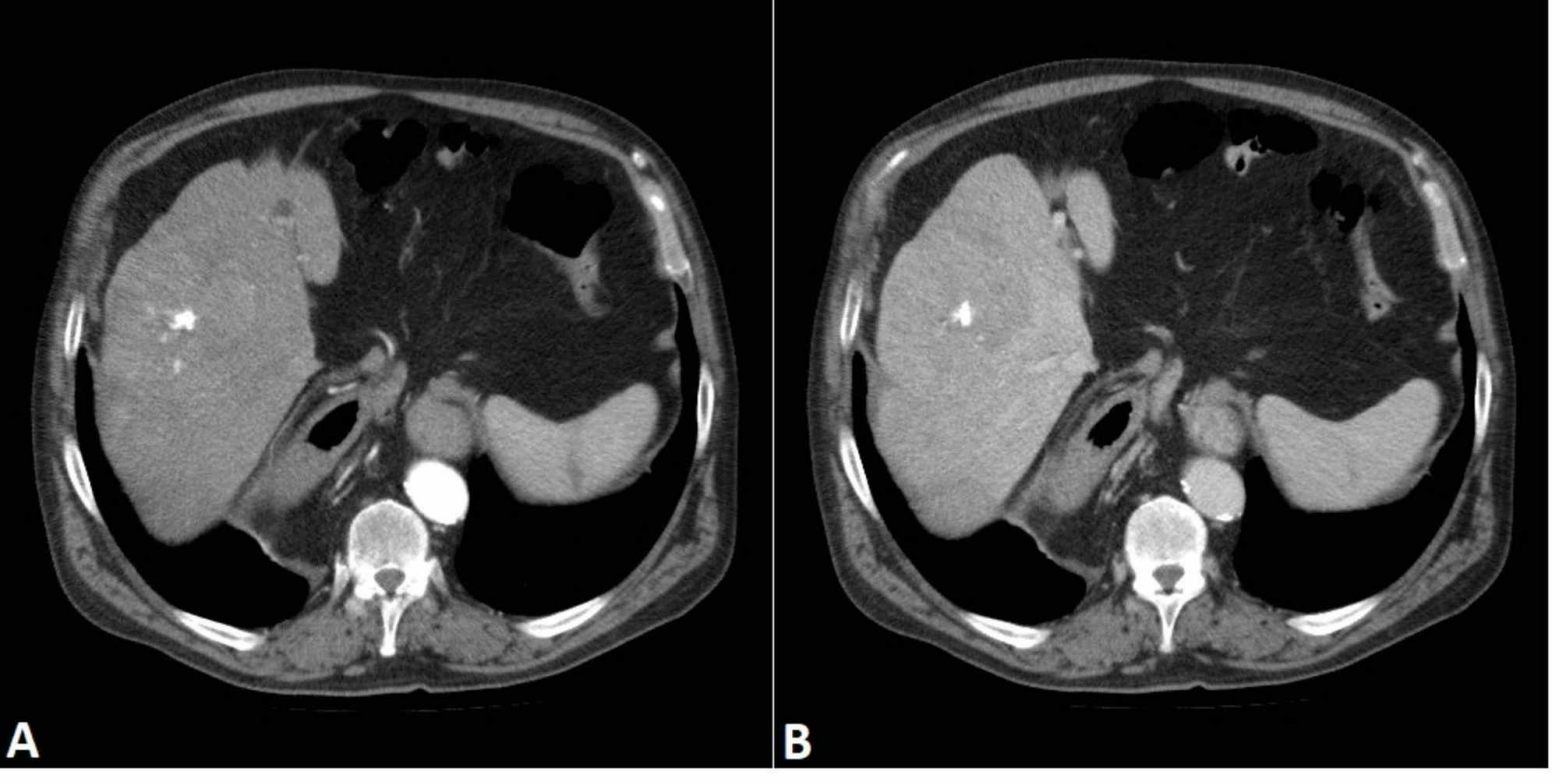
Liver mass. Follow-up CT examination shows enlargement of the main lesion (measuring 8 cm x 10 cm). The lesion has a calcified center with hyperdense septa spreading outwards. It demonstrates early enhancement in the arterial phase (A) with signs of quick wash-out in the portal phase (B).
